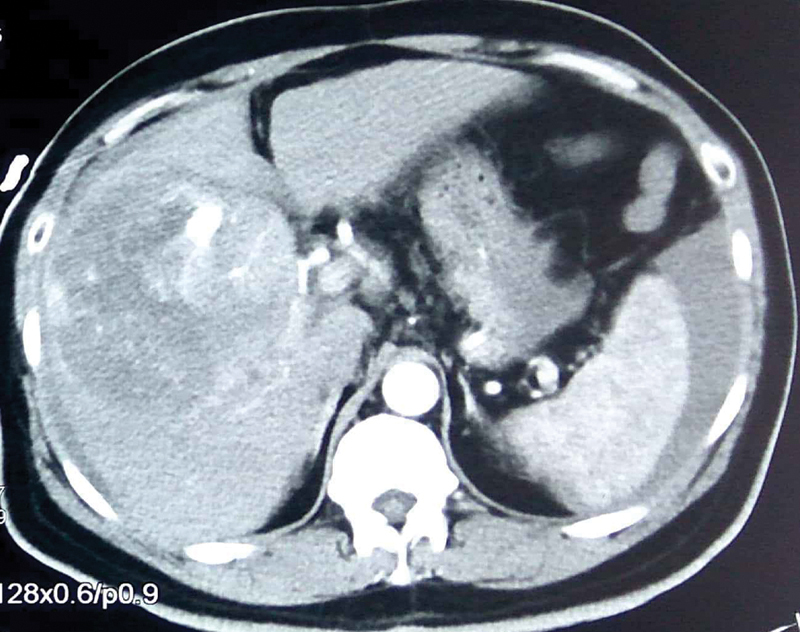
Contrast enhanced computed tomography axial image shows hemoperitoneum, arterially enhancing lesion in liver (hepatocellular carcinoma), and an intratumoral aneurysm with contrast blush.
